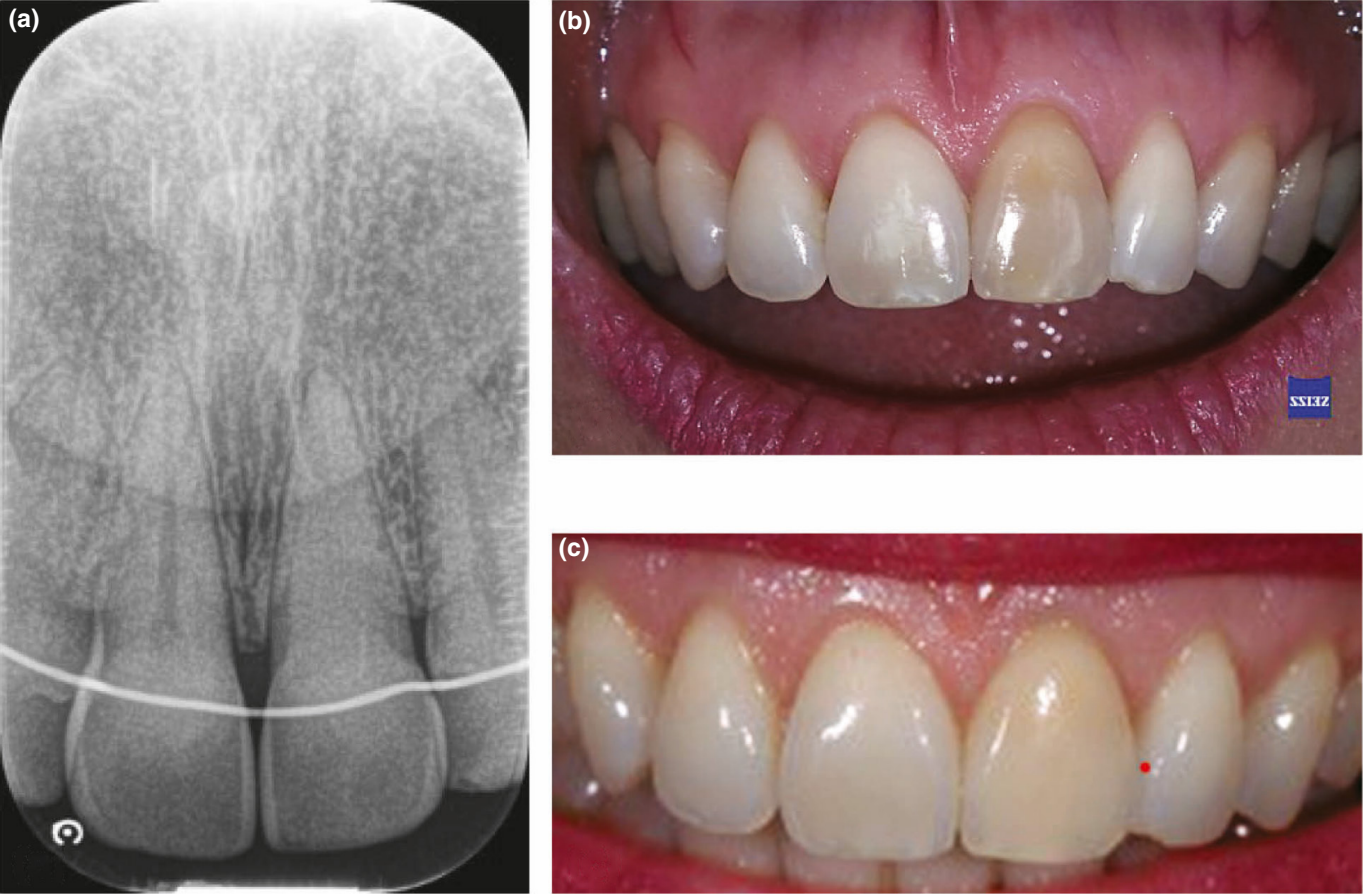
(a) A periapical radiograph of a left maxillary central incisor with extensive obliteration of the canal. The tooth was asymptomatic and nonresponsive to cold and electric pulp sensibility testing. Radiographically, there was no evidence of pathosis. (b) A preoperative photo showing discolouration. (c) The tooth was managed with external bleaching protocols using 6% hydrogen peroxide and customized trays (pola day/pola night; SDI Ltd.). The aesthetic outcome met the patient's expectation and improved the colour of all teeth in the smile zone. Courtesy of Dr Michael Lewis
